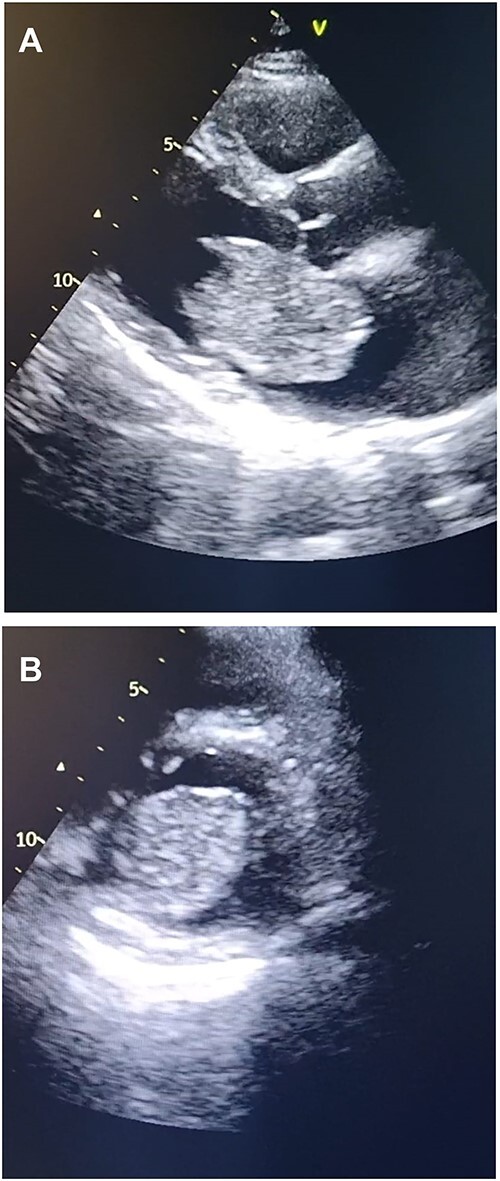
Transthoracic echocardiography. (A) Echocardiogram parasternal long-axis view (PLAX) view showing large homogenous mobile mass in LA. (B) Echocardiogram parasternal short-axis view (PSAX) view showing Left atrial mass protrusion through the Mitral valve during diastole.
